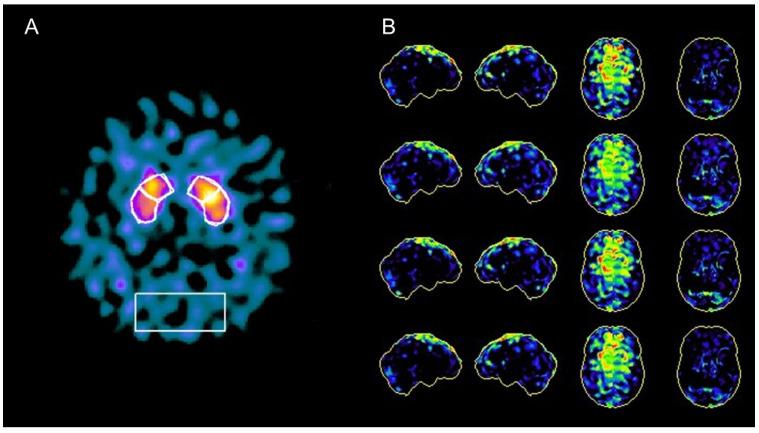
Nuclear imaging findings in a case of anti-IgLON5 disease: (A) [123I] FP-CIT SPECT showed dopaminergic nigrostriatal denervation, more prominent in the right putamen; (B) brain perfusion SPECT imaging documented relative hyperperfusion in the high-convexity area with relative preservation of other brain regions.
